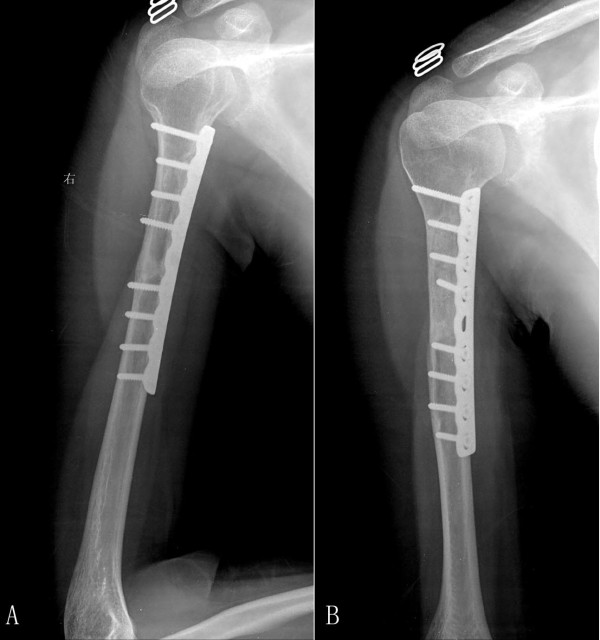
Radiographs at one year and 28 months after operation.
